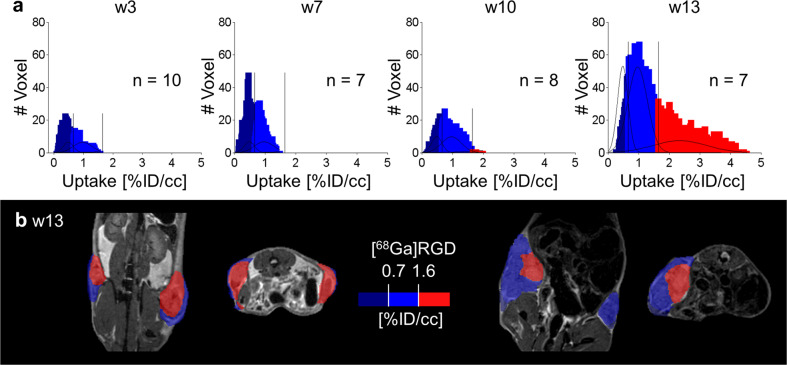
GMM analysis of [^68^Ga]RGD characterized IC regions. a The summed histogram of [^68^Ga]RGD uptake of all tumors and all time points was fitted and analyzed as described above for [^18^F]FDG, resulting in a sum of 3 Gaussian distributions as the best model. The thresholds 0.7 and 1.6%ID/cc were applied to the histograms of the single time points. b Representative PET/MR images revealed the highest uptake population (red, > 1.6%ID/cc) in only large tumors.
